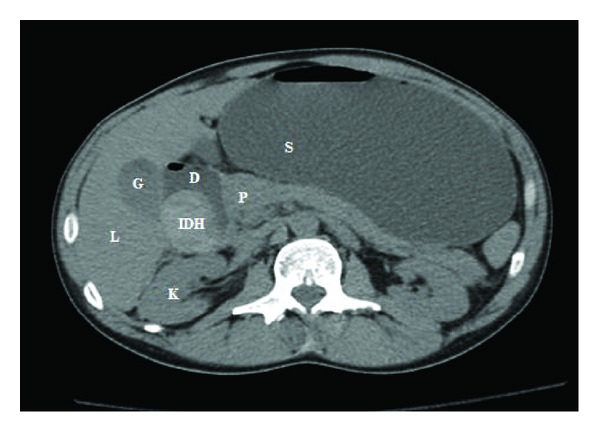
Axial image of noncontrast CT scan of the abdomen at the level of second part of duodenum demonstrates a circumferential hyperdense lesion in the wall of the second part of duodenum with peripancreatic fat stranding. IDH: intramural duodenal hematoma; D: second part of duodenum; P: pancreas; S: stomach; L: liver; K: kidney; G: gall bladder.
